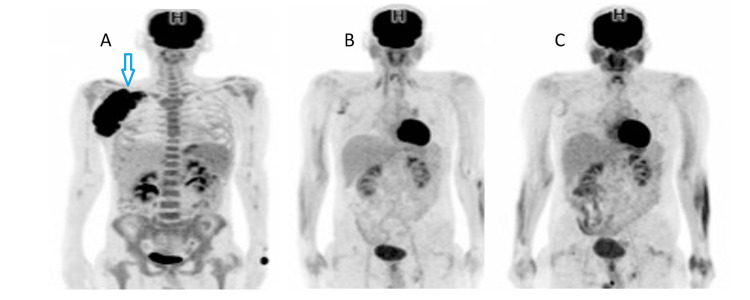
(A) A maximum intensity projection (MIP) PET scan at baseline demonstrates an intensely hypermetabolic conglomerate lymphadenopathy in the right axillary subpectoral region. (B) The MIP PET scan after two cycles of ABVD demonstrates a partial metabolic response with a dramatic regression of the intensely hypermetabolic lymphadenopathy but with mildly increased residual FDG uptake (Deauville 5PS=4). (C) The MIP PET scan after four cycles of ABVD demonstrates a complete metabolic response with minimal residual nonspecific FDG metabolic activity (Deauville 5PS=2). MIP: maximum intensity projection, PET: positron emission tomography, ABVD: doxorubicin, bleomycin, vincristine, and dacarbazine, Deauville 5PS: Deauville five-point scale, FDG: fludeoxyglucose F18
